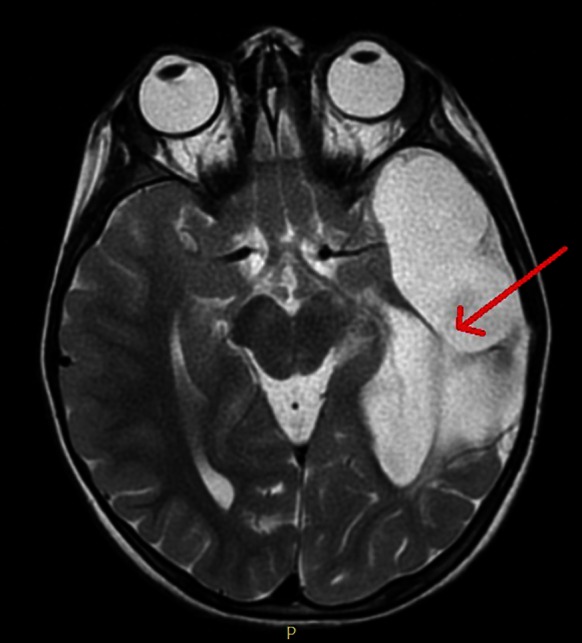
CT scan showing an arachnoid cyst (62 × 28 × 59 mm) between the temporal, parietal and frontal lobes
